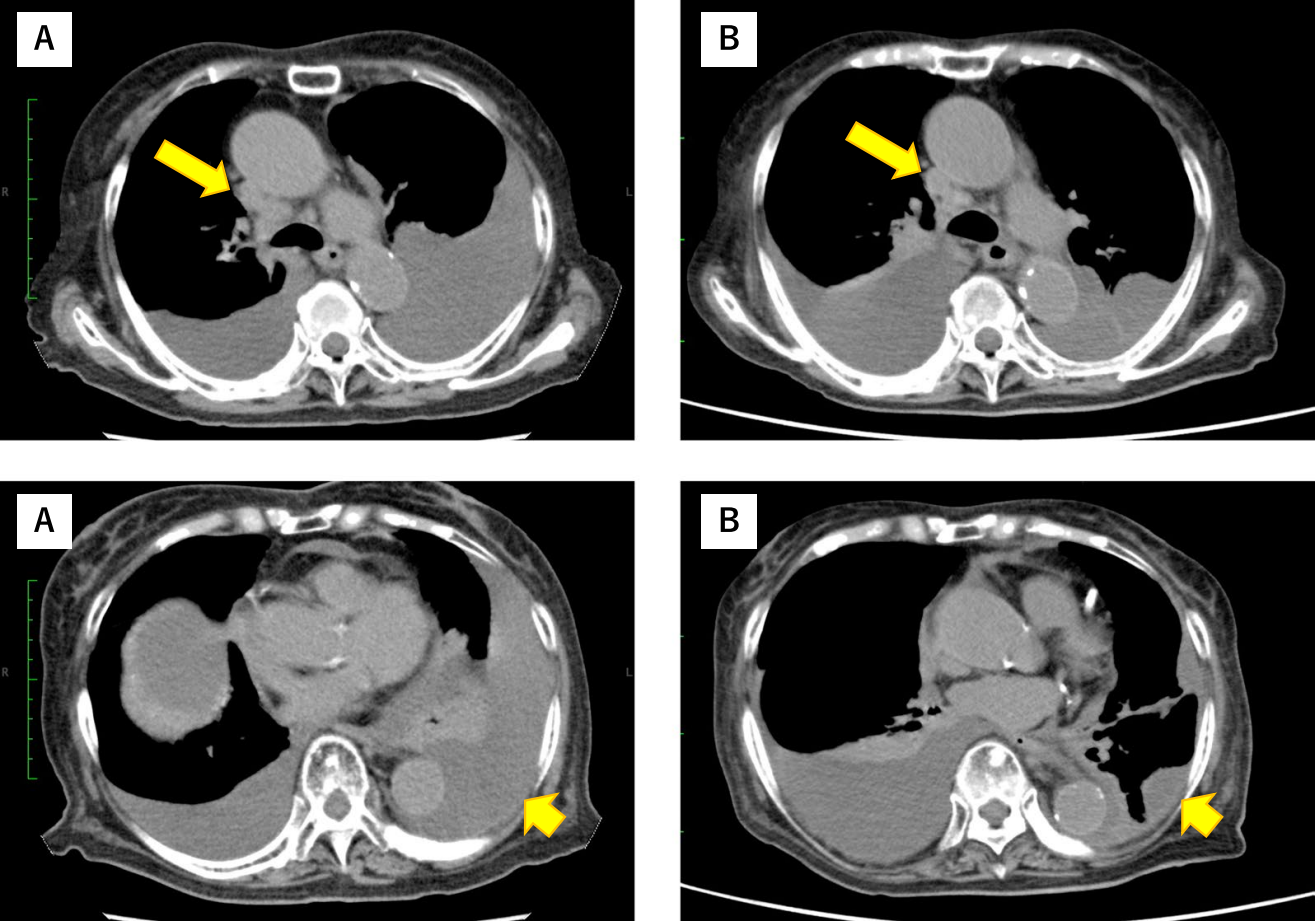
Chest computed tomography images before and after administering osimertinib. a Mediastinal lymph node metastasis and pleural effusions before treatment. b Slight tumor shrinkage in mediastinal lymph node metastasis (long arrow) and decrease in left pleural effusion (short arrow)
